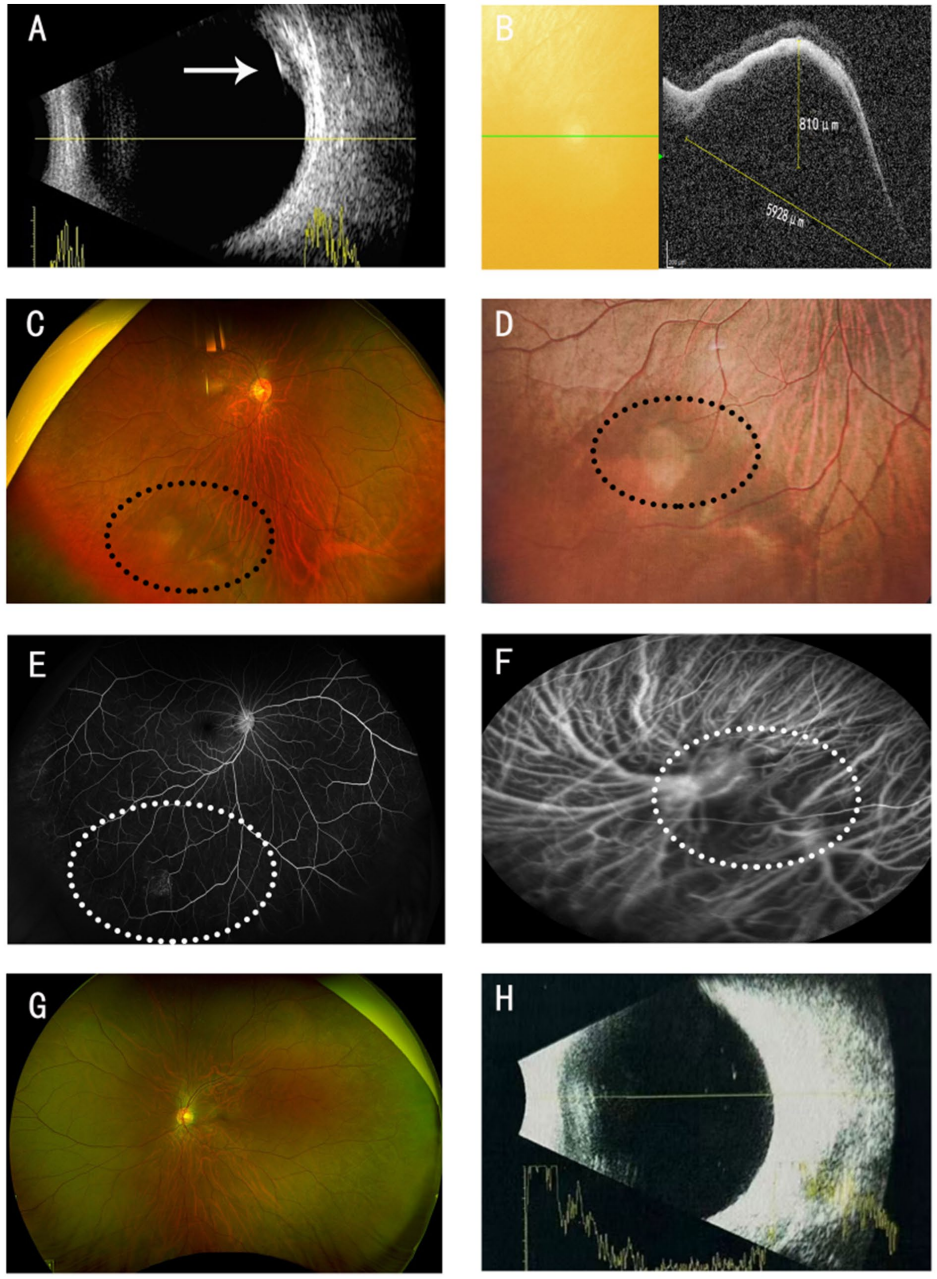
Right eye: (A) B-scan ultrasonography of the eye: Choroidal space-occupying lesion with a base diameter of 7.51 mm and a height of 2.51 mm, as indicated by the white arrow. (B) OCT: A low-reflective cavity with a height of 810 μm and a basal diameter of 5,928 μm is visible. (C,D) Fundus photography: A brownish-red elevated mass is visible in the inferotemporal region, as indicated by the black circle. (E) FFA: Localized fluorescein leakage is observed in the inferotemporal lesion, resulting in hyperfluorescence in the lesion area, as indicated by the white circle. (F) Dilation of the vortex vein ampulla, as indicated by the white circle. (G,H) Fundus photography, B-ultrasound: no abnormalities.
